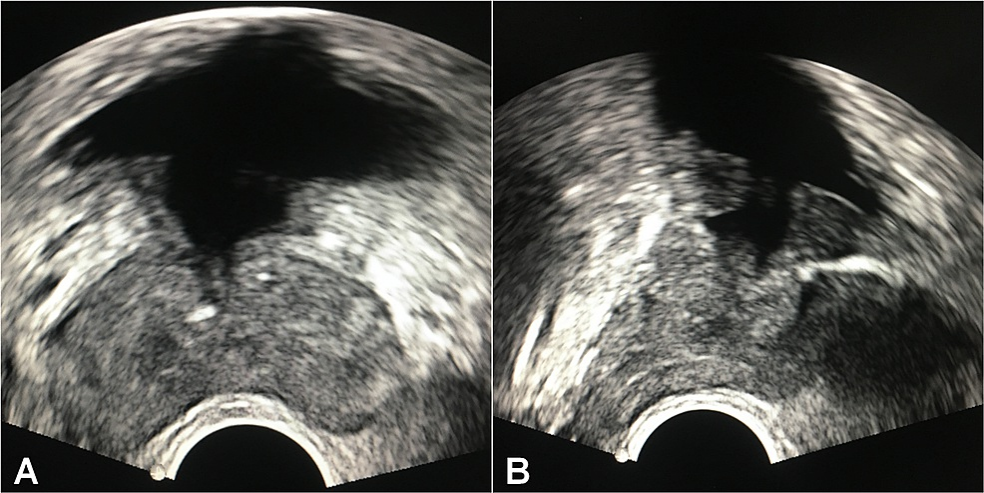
TRUS image showing a residual cystic cavity at the bladder neck region. (A) Axial TRUS image. (B) Sagittal TRUS image. TRUS:  transrectal ultrasonography
